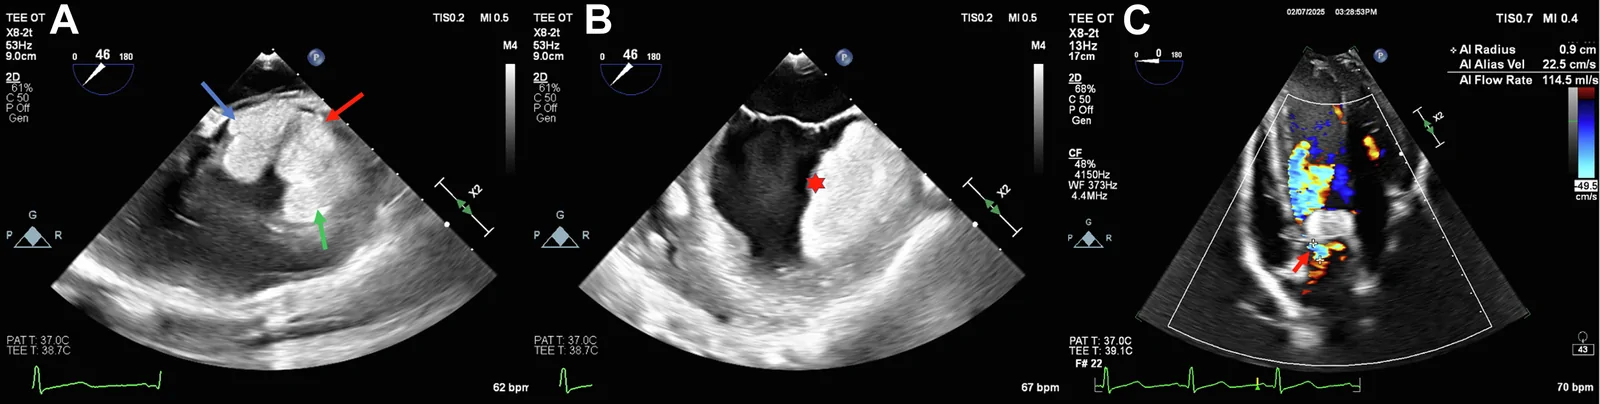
2D Transesophageal Echocardiography (TEE) and Color Flow Doppler Across Aortic Valve (A) Transesophageal echocardiogram mid-esophageal short-axis view showing masses at the noncoronary cusp (blue arrow), left coronary cusp (red arrow), and left ventricular wall mass (green arrow). (B) Mid-esophageal view demonstrating left ventricular wall mass. (C) Color Doppler from a deep transgastric view showing severe aortic regurgitation. AI = aortic insufficiency; CF = colour flow; MI = myocardial infarction; PAT = patient's temperature; TEE OT = transesophageal echocardiography operating theater; TIS = tissue imaging system.
